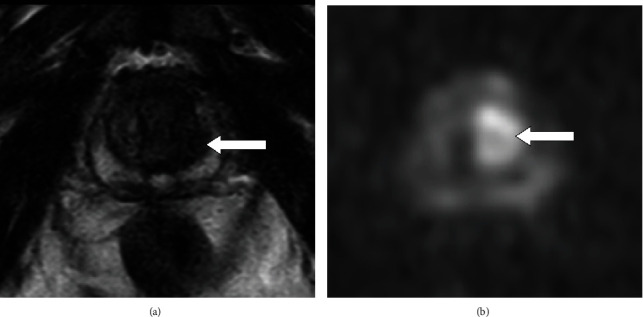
(a) Magnetic resonance imaging showed a low-intensity area in the T2-weighted image (white arrow). (b) A high-intensity area in the diffusion-weighted image at the left transition zone (white arrow).
